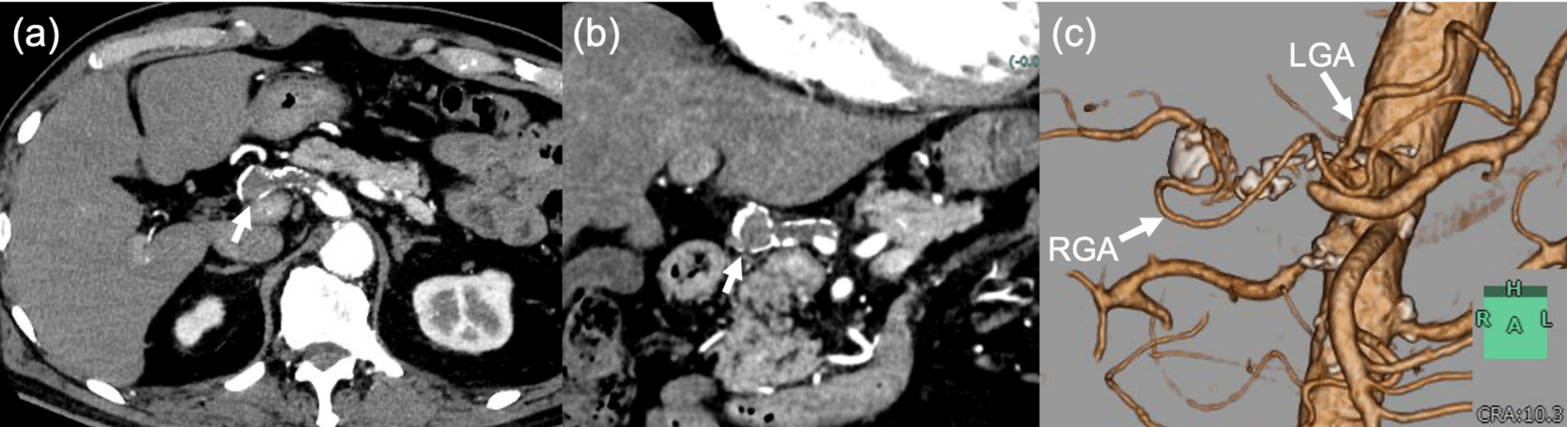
Long-term follow-up contrast-enhanced CT at 11 years (a) Axial arterial-phase contrast-enhanced CT image. (b) Coronal arterial-phase contrast-enhanced CT image. (c) Volume-rendered three-dimensional reconstruction. At 11 years after the initial diagnosis, persistent complete thrombosis of the hepatic artery aneurysm is confirmed (arrows), with no evidence of recanalization, enlargement, or secondary complications. On volume-rendered imaging, the collateral pathway from the left gastric artery to the right gastric artery is clearly visualized and appears more prominent than at the initial examination.
